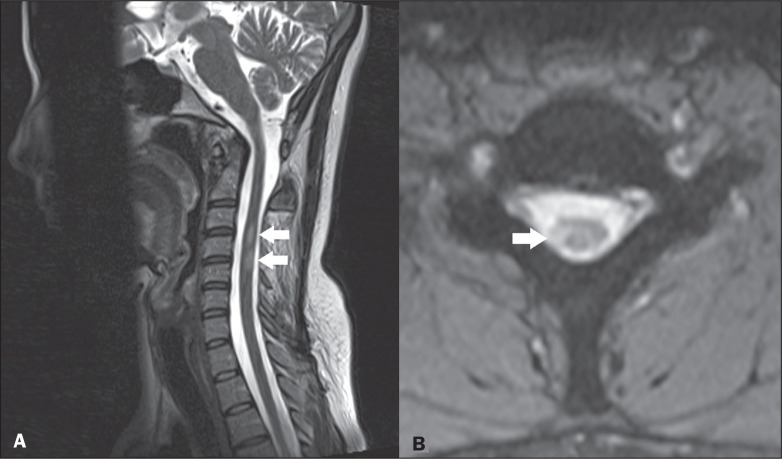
Multiple sclerosis. Sagittal T2-weighted and axial T2*-weighted MRI
